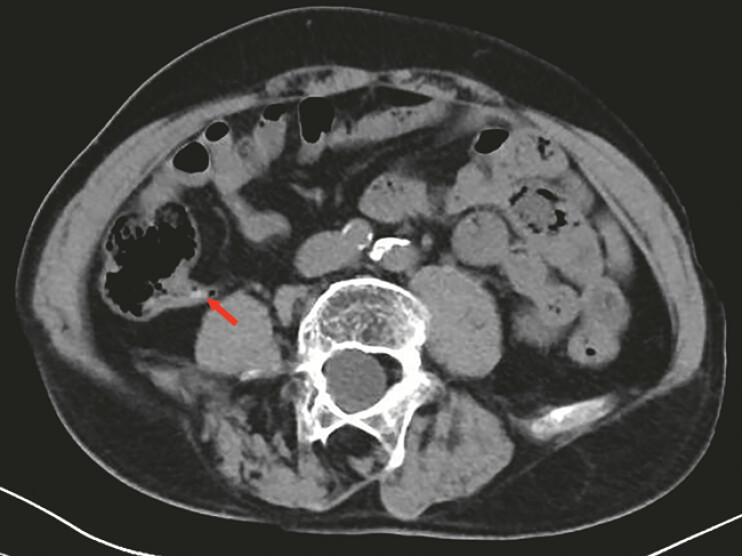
The abdominal CT revealed appendicitis and appendiceal fecalith (the red arrow).
